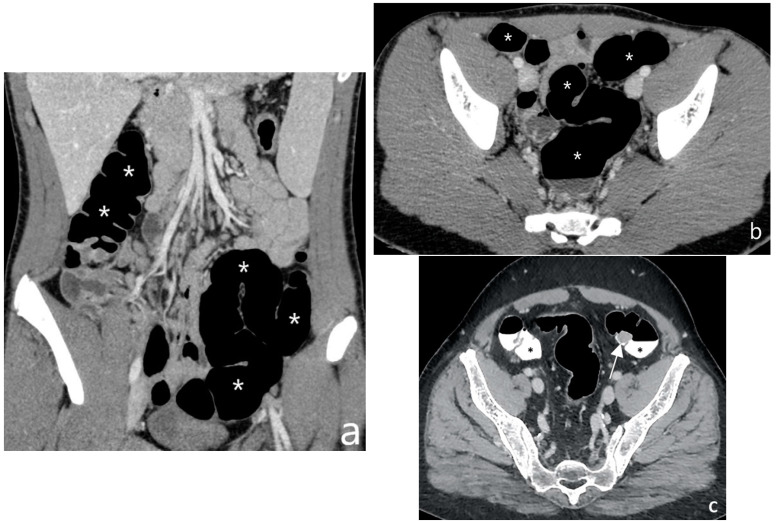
CT after iodinated contrast injection: example of colon distension using air by endorectal insufflation in the coronal (a) and axial (b) planes (asterisks). (c) Another example of colonic distension using air by endorectal insufflation in the axial plane shows a polypoid thickening of the sigmoid colon (arrow); hyperdense contrast agent for faecal tagging is also evident in the lumen (black asterisks). Original figures by LMM, LL and BB.
